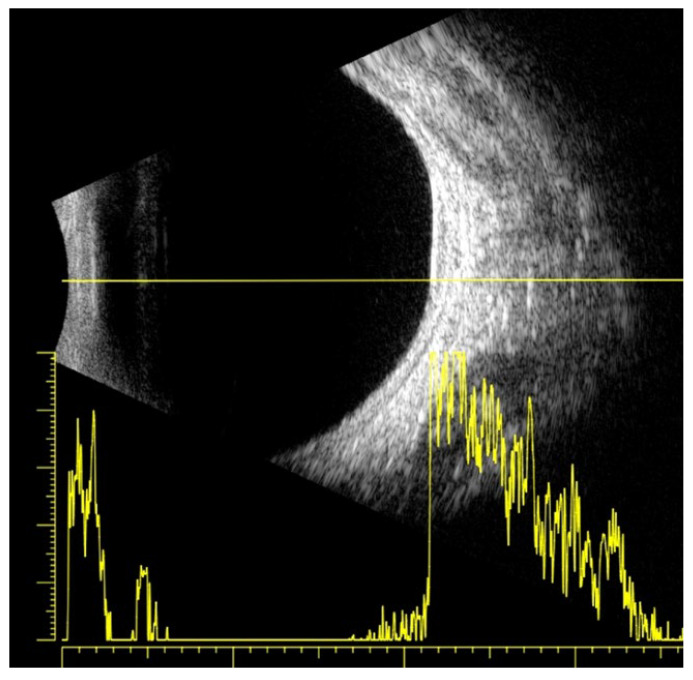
A-mode and B-mode ultrasonography of the choroidal nevus.
